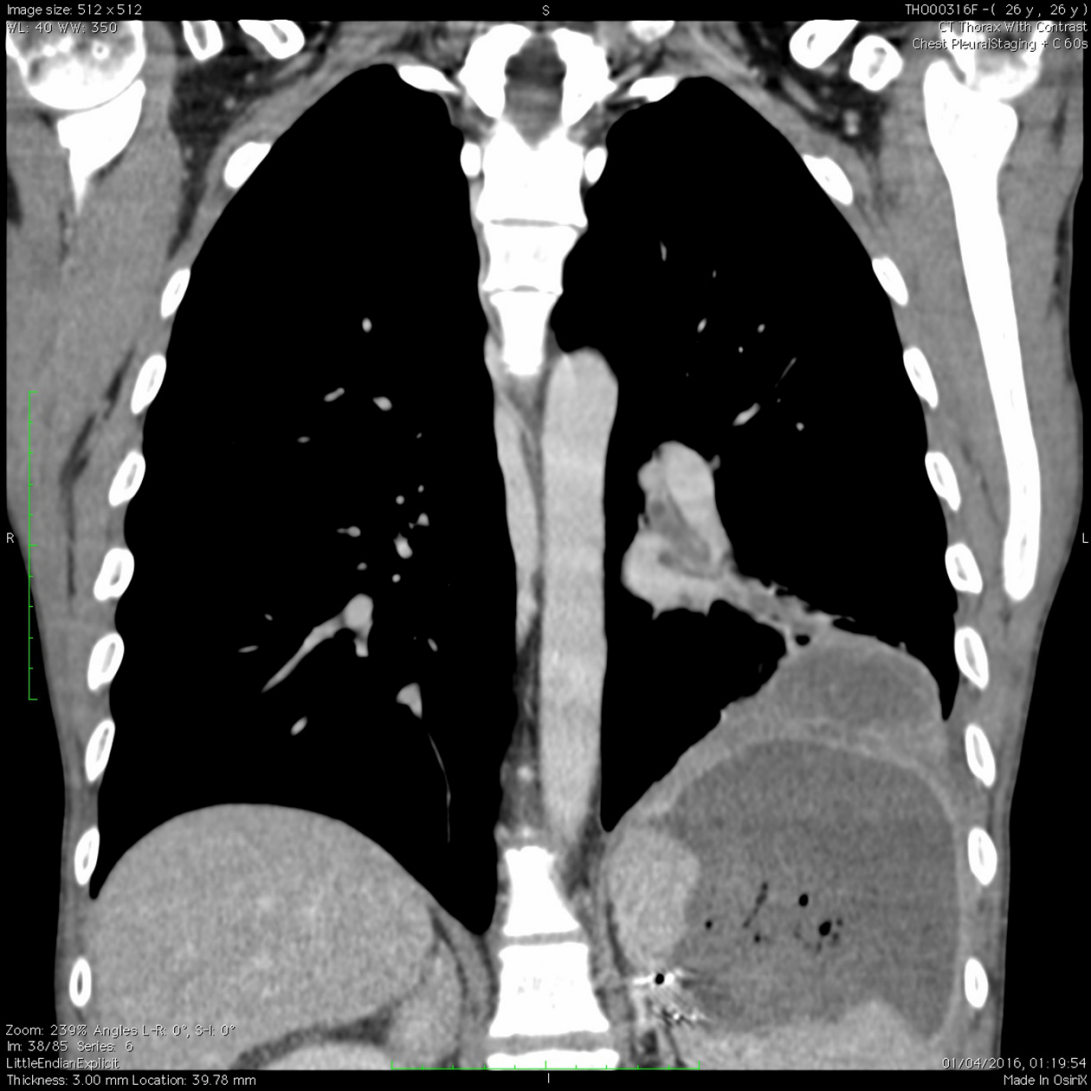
Coronal view of CT scan of abdomen/pelvis with contrast, taken at 60s with Omnipaque 350 agent delivered at 3 ml/s. Demonstration of the splenobronchial fistula; coursing from the spleen via the abscess, into the medial left bronchial region.
